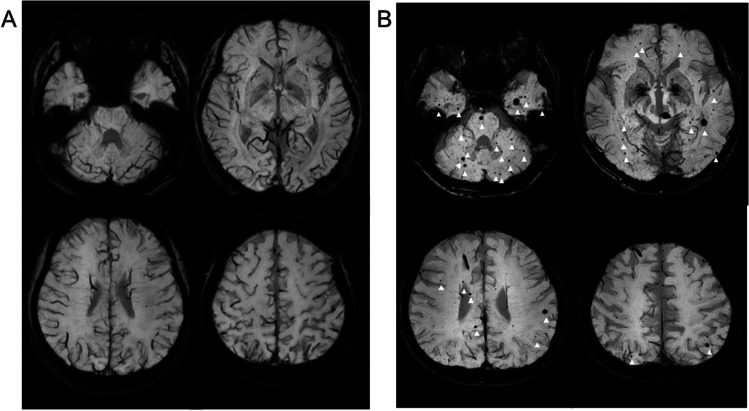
Susceptibility-weighted magnetic resonance imaging (SWI) of two patients with germinoma. A The representative patient without microbleeds. A 25-year-old male patient did not have any CMBs on SWI at 118 months after treatment. He was diagnosed with neurohypophyseal germinoma at the age of 15 years and was treated with cisplatin/etoposide and carboplatin/etoposide, followed by radiation therapy to the whole ventricle at a dose of 24 Gy. B The representative patient with microbleeds. A 40-year-old male patient had 41 CMBs in the cerebrum, basal ganglia, brain stem, and cerebellum on SWI at 317 months after treatment. He had pineal germinoma at the age of 14 years and was treated with radiation therapy to the whole brain at a dose of 30 Gy and to the primary site at a dose of 20 Gy. CMBs with more than 2 mm diameter are indicated by arrowheads
